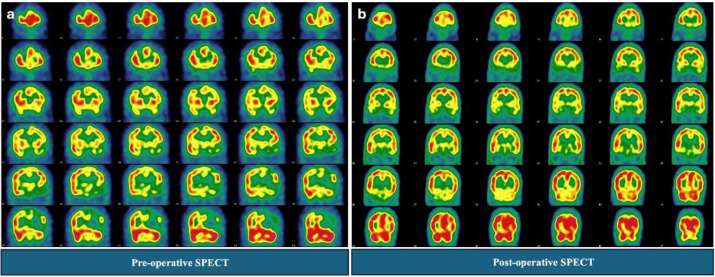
17 years old patient presented with multiple regions of Type II and Type III CVR response in the left hemisphere pre operatively (a). Follow-up SPECT performed six months after surgery demonstrated a significant improvement in perfusion to the previously compromised territories, which now showed a normal Type I response (b), consistent with successful revascularization and a good clinical outcome
